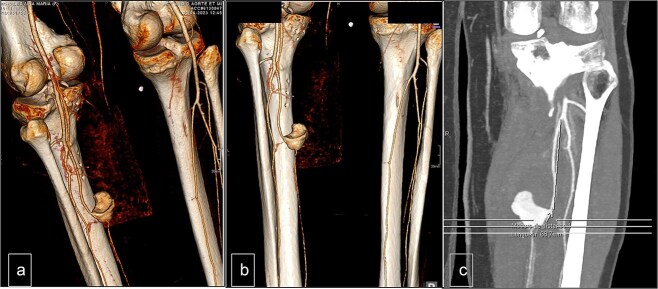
Computed tomography. (a) Lateral view, (b) posterior view, and (c) vascular reconstruction. The medial wall of the posterior tibial artery appeared discontinuous over a length of ~6 mm.
